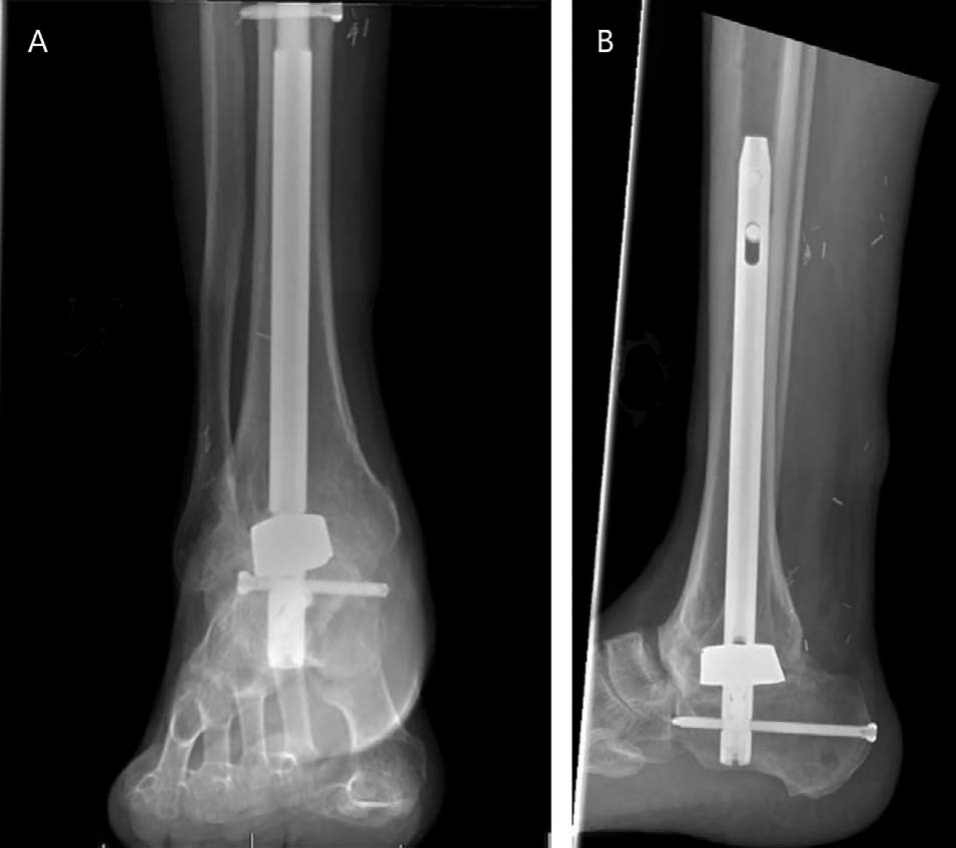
(A) Anteroposterior and (B) lateral x-rays of the patient's right ankle 2 years after hindfoot fusion show complete fusion of the tibia and calcaneus.
